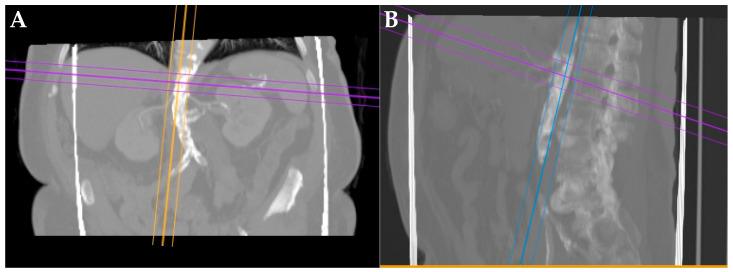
(A) Preoperative coronal and (B) sagittal computed tomography angiography (CTA) images showing severe narrowing of the abdominal aorta consistent with midaortic syndrome. Multiplanar reconstruction lines indicate the planes selected for further evaluation and planning of endovascular chimney repair.
